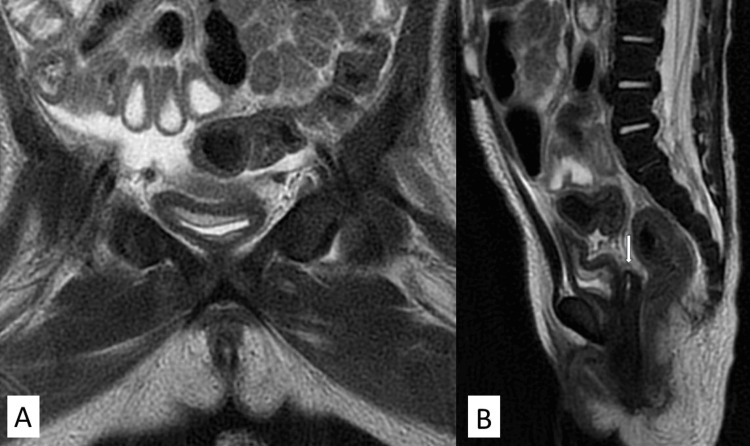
MRI. A: Coronal TWI demonstrates no ovaries; B: sagittal T2: Small blind-ended  tubular structure noted posterior to the urethra and anterior to the rectum most likely representing the vestigial vagina MRI : Magnetic resonance imaging; TWI: track weighted imaging
